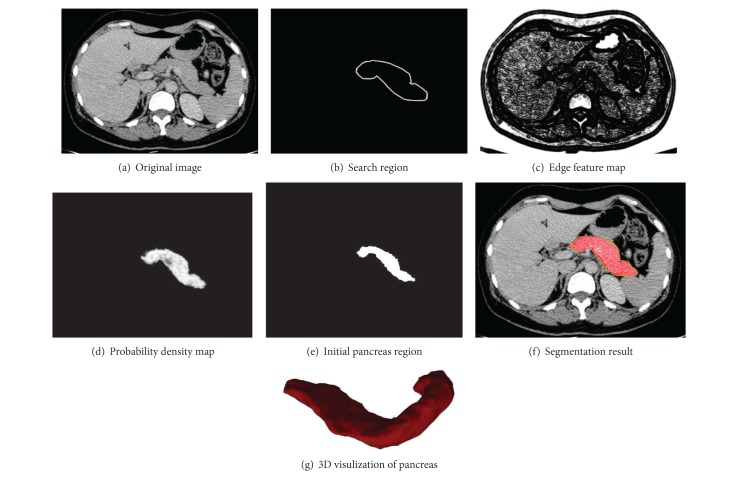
Segmentation example: necessary reprocessing for pancreas segmentation. (a) Denoised CT image. (b) The mask of previous slice. (c) The search region of pancreas based on mask of previous slice. (d) Edge feature map. (e) Probability density map. (f) Initial pancreas region used in our level set method.
